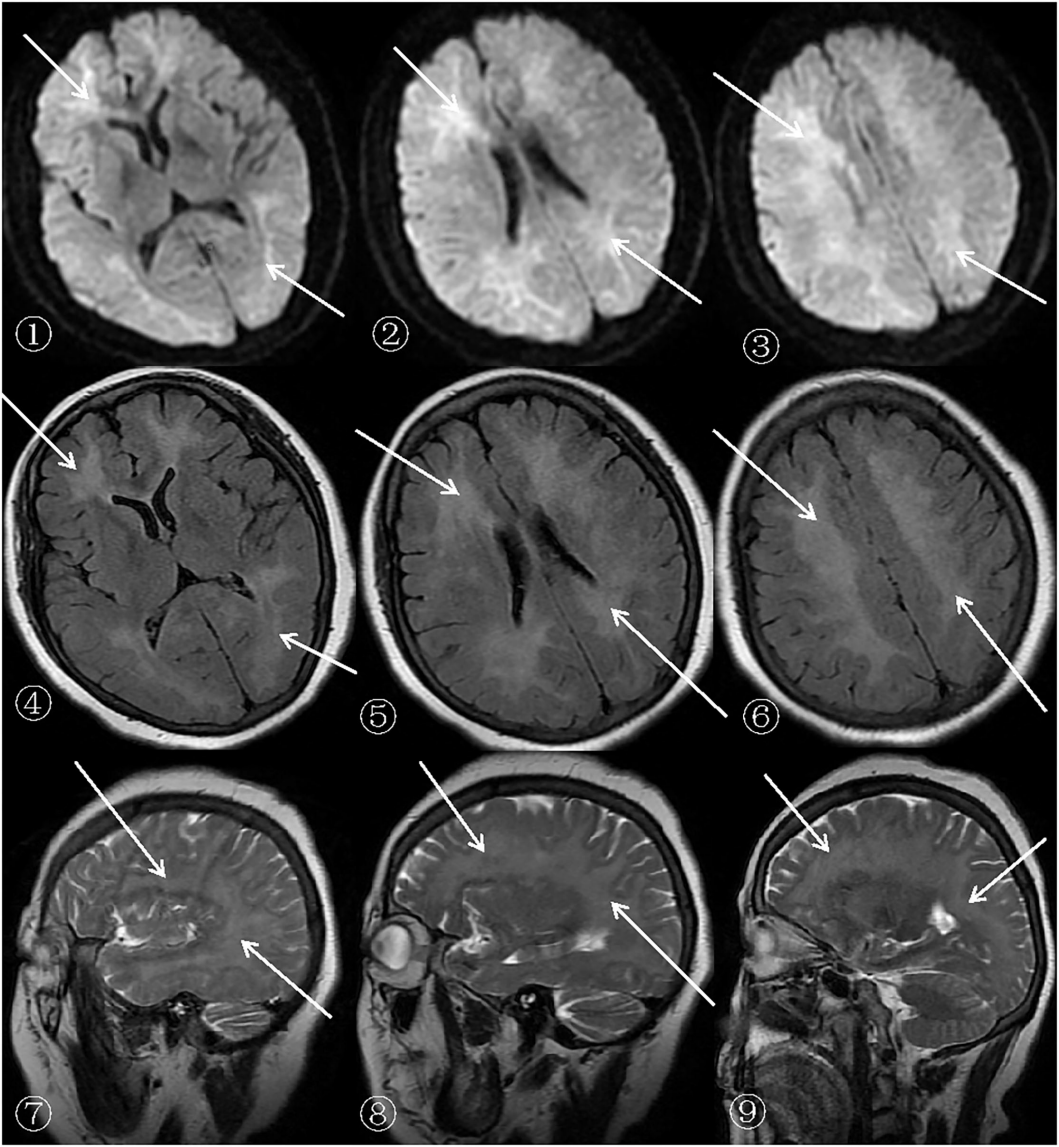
MRI (SIEMENSAGFWB, skyra 3.0 tesla) images of the patient's brain.
